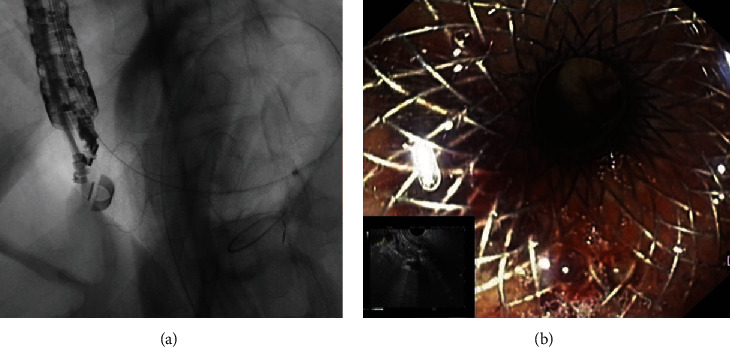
Patient with a previous Roux-en-Y gastric bypass who presented with jaundice secondary to pancreatic cancer underwent endoscopic ultrasound directed transgastric ERCP (EDGE). (a) Fluoroscopic image showing a 20 mm diameter lumen apposing metal stent (LAMS) placed between the gastric pouch and the gastric remnant under EUS guidance. (b) Endoscopic image of the proximal flange of the LAMS in the gastric pouch.
